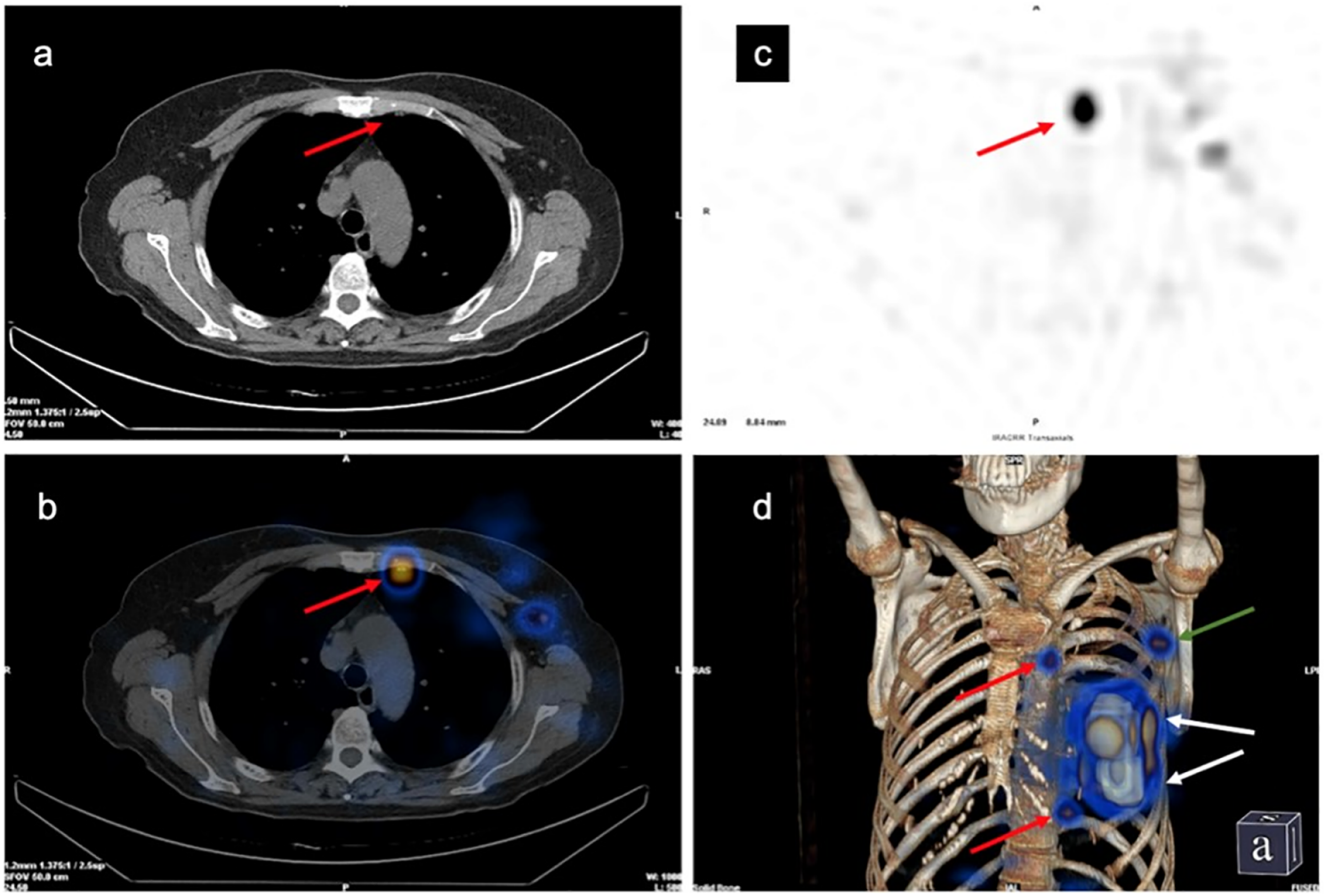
Development of the left internal mammary sentinel lymph nodes (IM-SLN) and left axillary sentinel lymph node (A-SLN). (A) Computed tomography (CT) plain scan. (B) Single-photon emission computed tomography imaging. (C) Tomographic fusion image. (D) 3D image. Red arrows, IM-SLNs; white arrows, injection points; green arrow, A-SLN.
